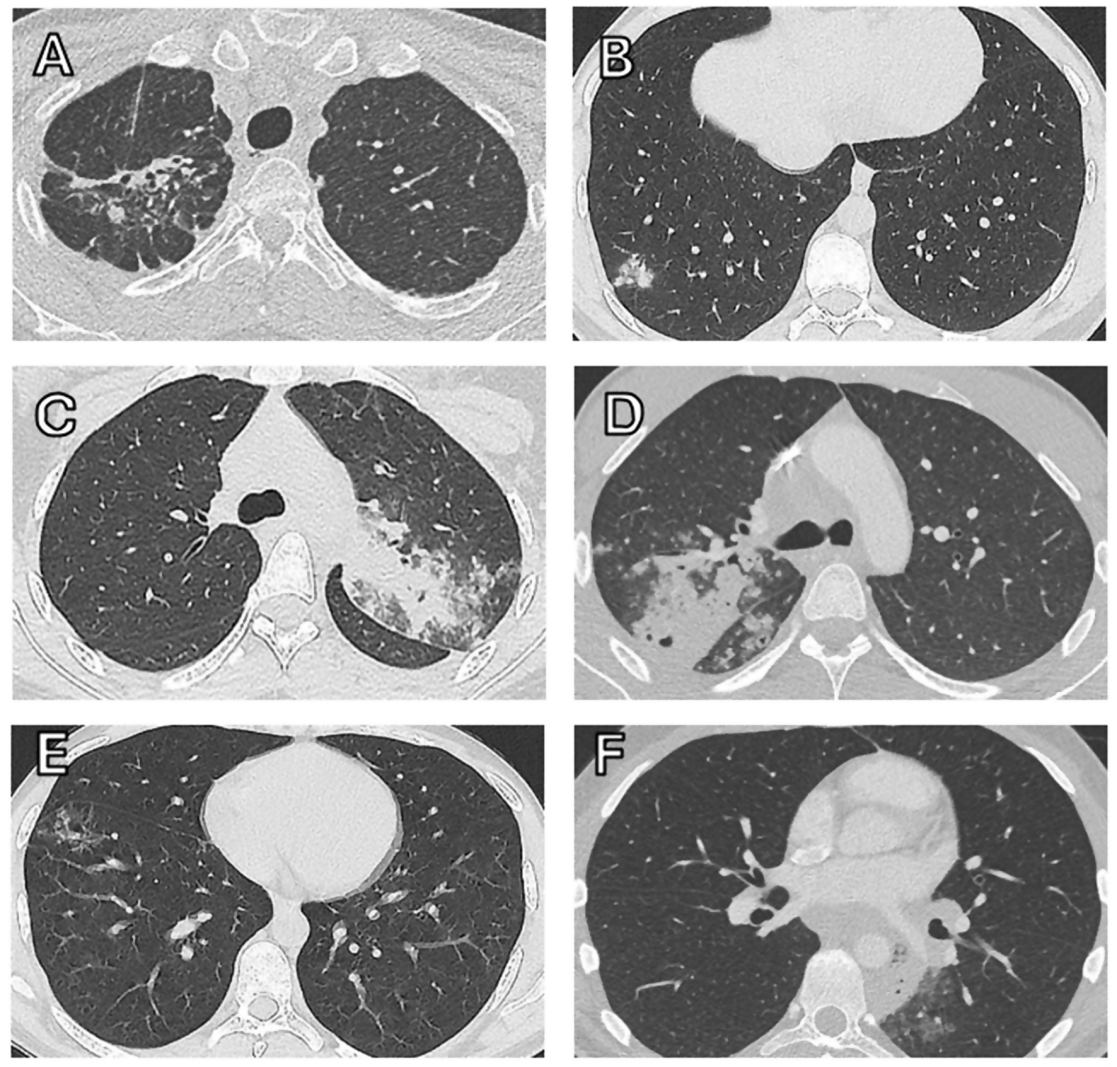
Chest CT scans of patients 1 to 6. Legend: A: Patient 1; B: Patient 2; C: Patient 3 D: Patient 4; E: Patient 5; F: Patient 6
